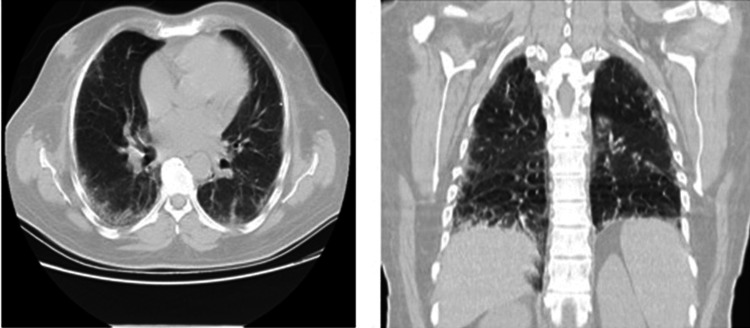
Chest CT scan left: horizontal section; right: frontal section
